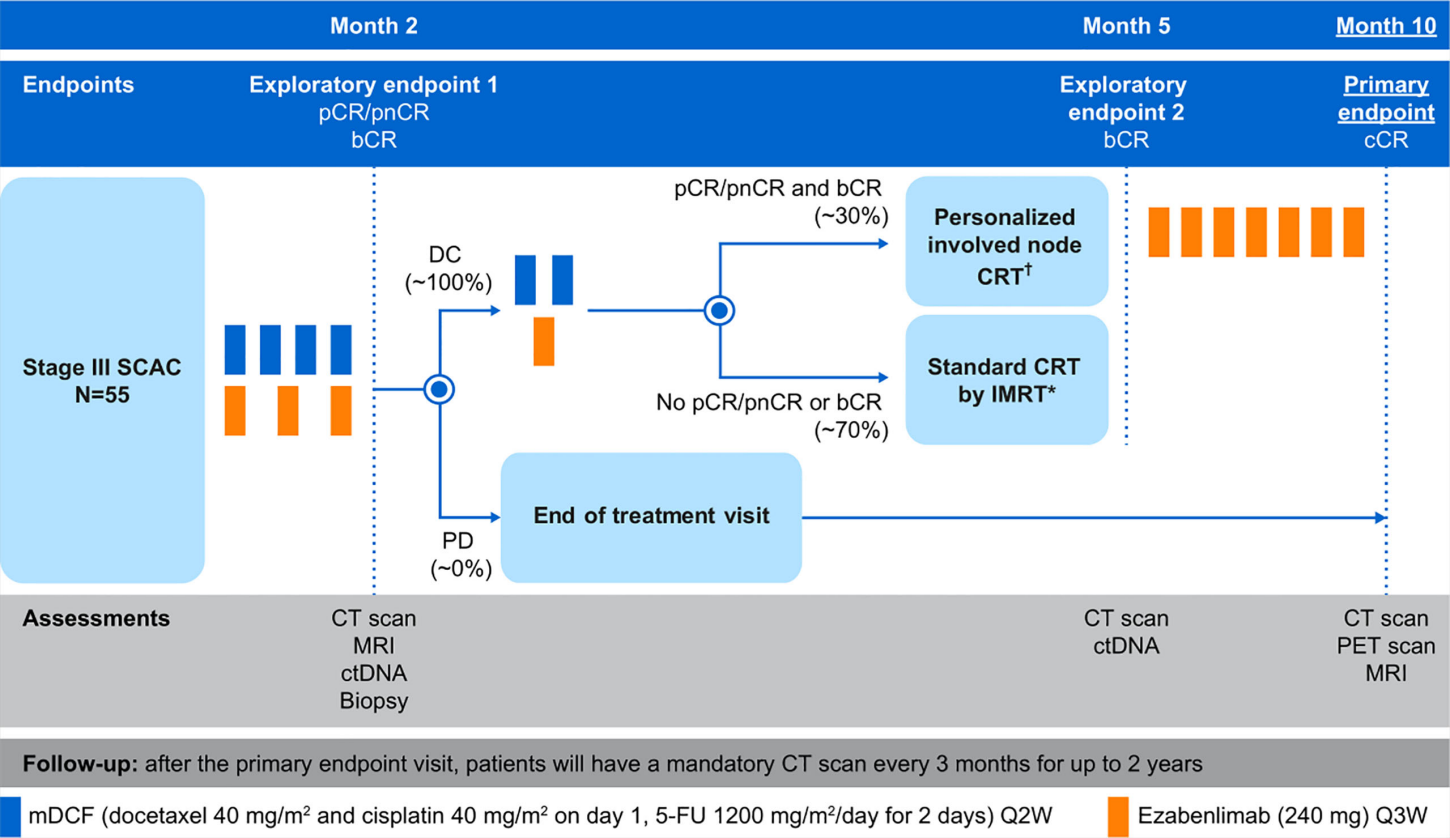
Study Design of INTERACT-ION. *Concomitantly given with capecitabine (825 mg/m^2^ orally twice daily) only on days of radiotherapy, and mitomycin C (10 mg/m^2^ Day 1 ± Day 29). ^†^59.4 Gy for primary tumor and involved residual nodes + 45 Gy for elective nodal irradiation for initially involved lymph node(s) with complete response. 5-FU, 5-fluorouracil; bCR, biological complete response; cCR, complete clinical response; CT, computed tomography; ctDNA, circulating tumor DNA; DC, disease control; IMRT, intensity-modulated radiation therapy; MRI, magnetic resonance imaging; pCR, pathological complete response; PD, progressive disease; PET, positron emission tomography; pnCR, pathological near-complete response; Q2W, every 2 weeks.
